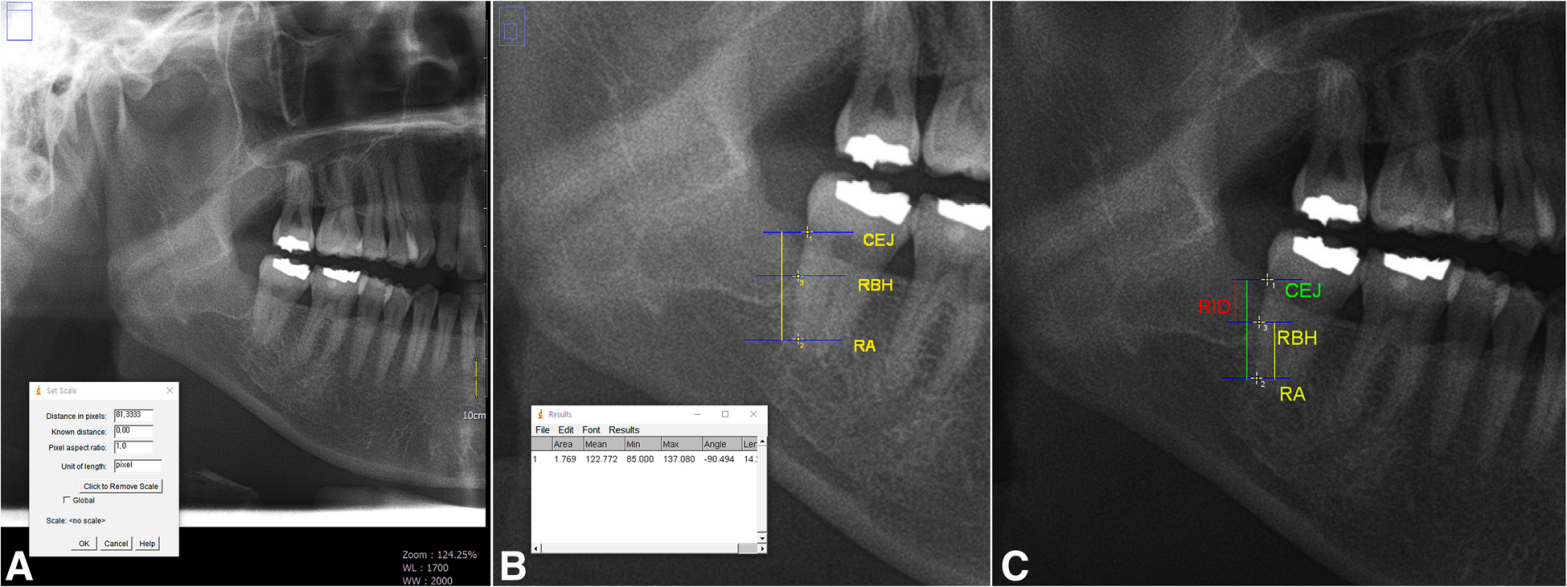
Panoramic radiograph screening using imageJ® software. a The process of setting the distance per pixel unit using the scale tool in the ImageJ® software and the ruler in the panoramic image. b The process of measuring the desired linear distance in the region of interest (ROI) using the set scale value. c Reference points and radiographic variables: (1) CEJ; (2) root apex of the distal root (RA); (3) Uppermost intersecting point between distal root of M2 and mesial wall of extraction socket; RBH, Radiographic bone height (yellow line); distance between CEJ and RA (green line); RID, Radiographic infrabony defect (red line)
